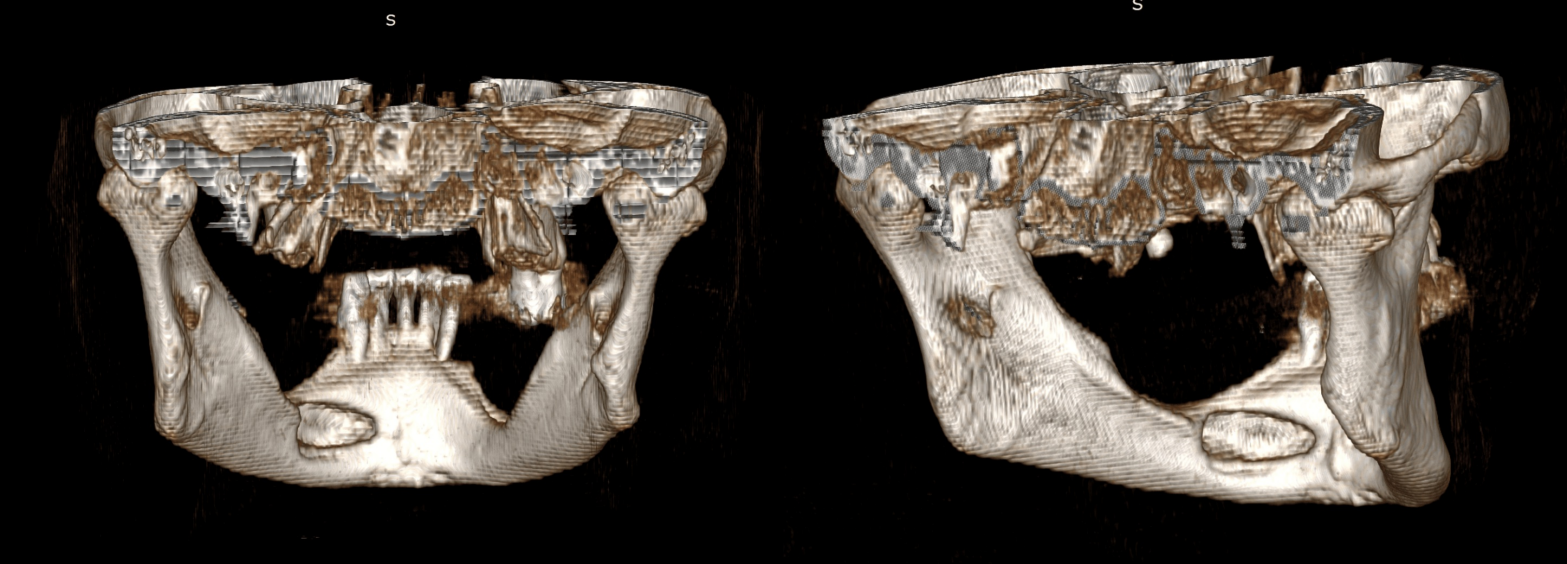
Three-dimensional model created from CT data. Left: Anterior view of the mandible. Right: Left anterior view of the mandible.
